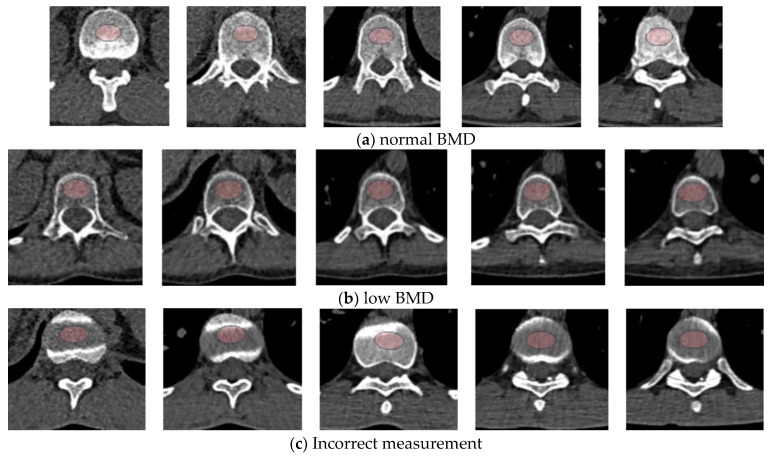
Examples of AI-assisted determinations of attenuation values for vertebral bodies L1 to Th9 using low-dose computed tomography (LDCT). From left to right: L1–Th9. (a) Normal findings. The ROI placed by the AI was appropriately centered on the vertebral body to be measured. The measured HU values ranged from 223 to 364. (b) Low BMD: The ROI is centered correctly by the AI on the vertebral bodies for the assessment of attenuation values. This results in HU values ranging between 89 and 117. (c) Incorrect measurements: The ROIs for L1, Th12, Th10, and Th9 were placed within the intervertebral disc space, resulting in underscored HU measurements for L1 (89), Th12 (122), Th10 (88), and Th9 (94). Conversely, the ROI for Th11 was positioned centrally within the vertebral body, with an HU value of 255. AI, artificial intelligence; ROI, region of interest; HU, Hounsfield unit; BMD, bone mass density.
